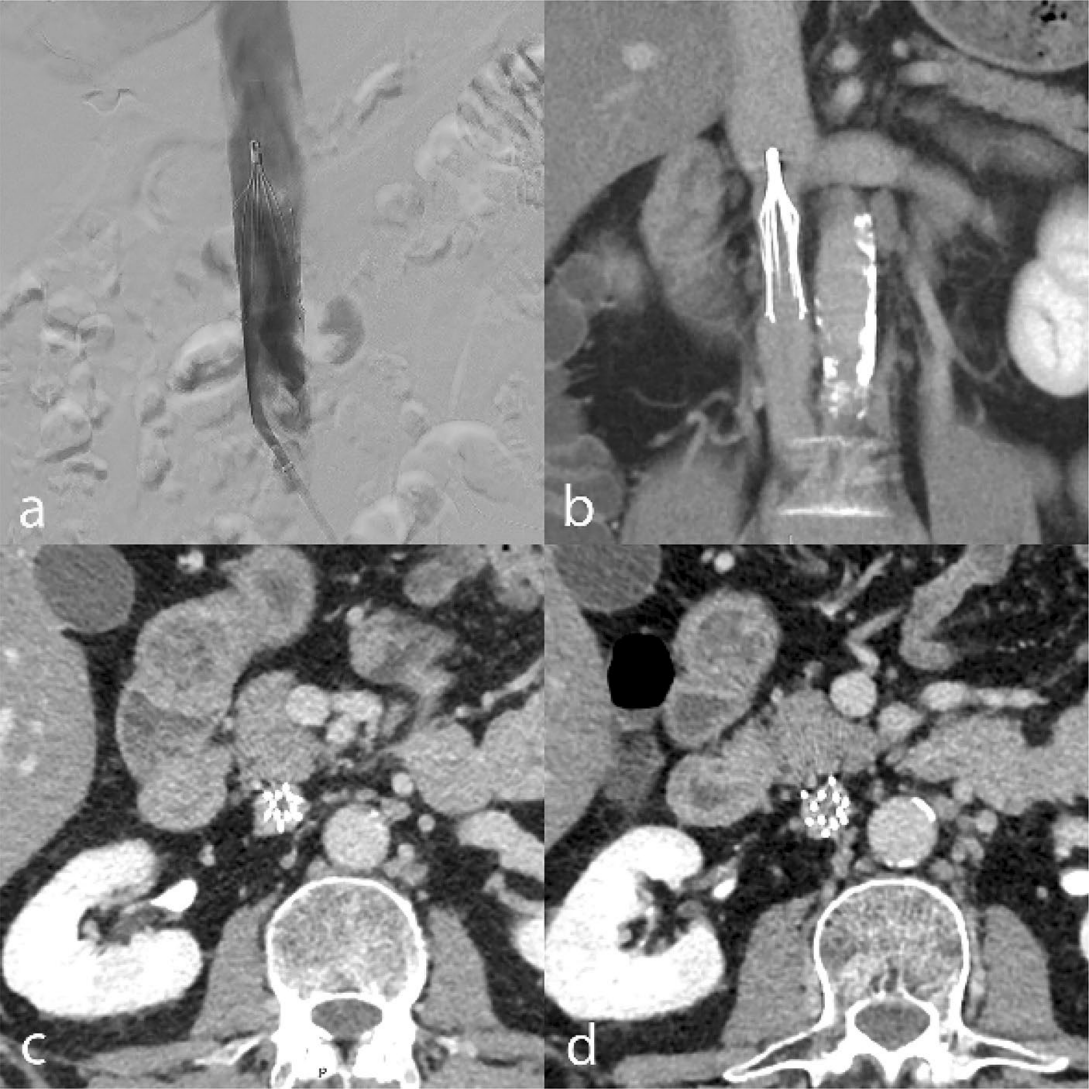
Post-deployment subtracted cavography shows IVCF positioned in infrarenal position and absence of IVC stenosis, in a patient with femoral and iliac DVT under ACT (a); CT venography obtained 5 months later shows IVC stenosis on coronal reformatted image (b), and thrombus trapped inside the IVCF (c,d), leading to technical ineligibility for retrieval. *ACT* anticoagulation therapy, *CT* computed tomography, *DVT* deep venous thrombosis, *IVC* inferior vena cava, *IVCF* inferior vena cava filter.
